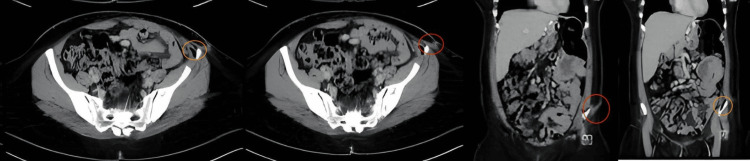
Axial and coronal CT images of the pelvis and abdomen reveal a tract extending from the subcutaneous tissue and skin into the left iliacus muscle at the level of the iliac bone (red circle). A small air locule is seen at the donor site, interposed between the iliacus muscle and iliac bone, without extension into the abdominal or pelvic cavity (orange circle). CT, computed tomography
